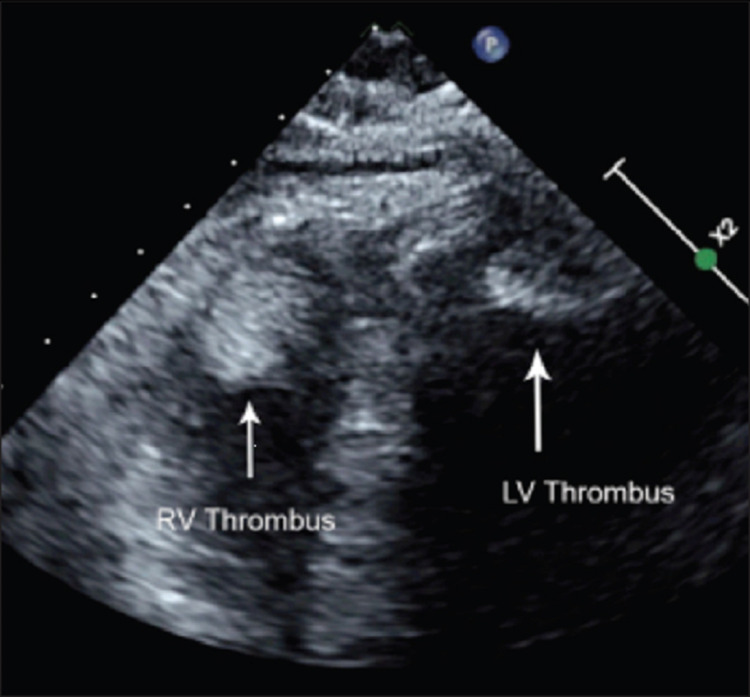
Echocardiogram shows the thrombus visible in right and left ventricles (white arrow) LV: left ventricular; RV: right ventricular
